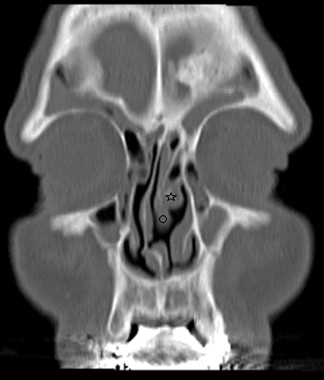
Coronal paranasal computed tomography slice showing overextension of superior turbinate toward the inferior turbinate on the left and a severe nasal septum deviation on the right. Also sinusitis in both maxillary sinuses is seen (☆: the middle turbinate, ◯: the superior turbinate).
